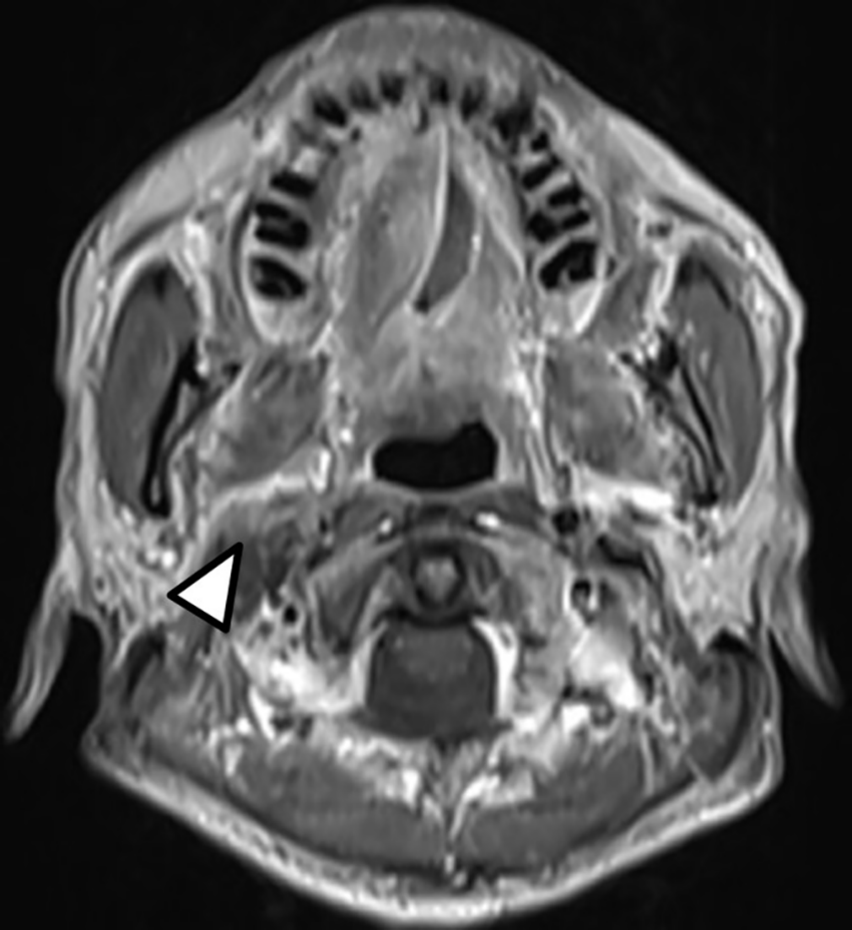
Post-treatment T1-weighted head and neck MRI showed decreased size of the right carotid body tumor with slightly anterior displacement of the right parapharyngeal fat (Arrow head).
